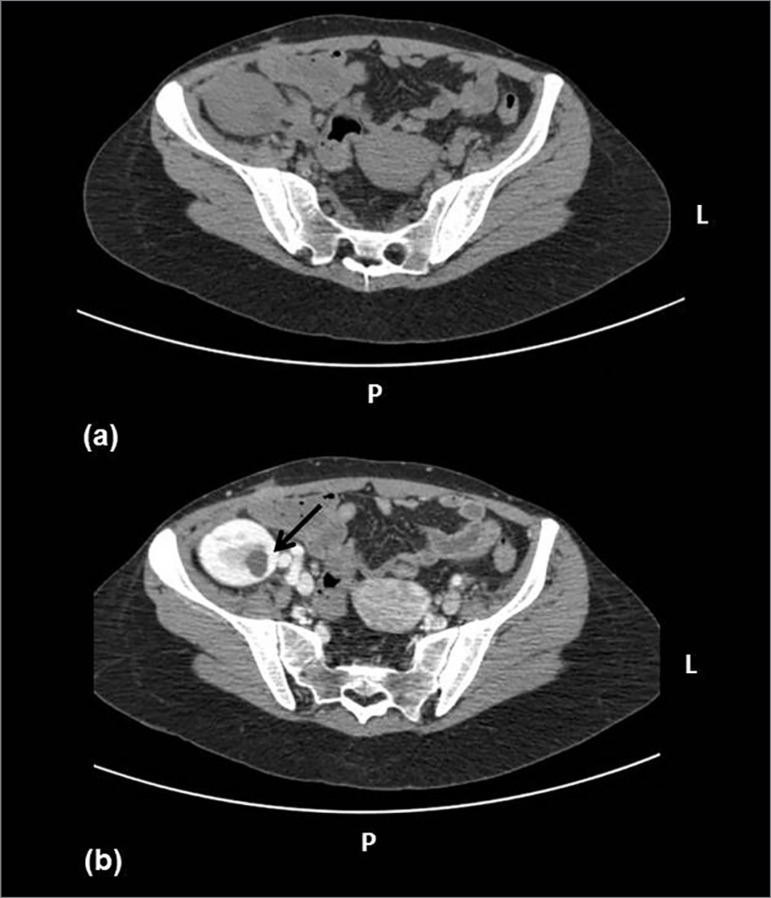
Panel A. Non-enhanced CT scan showing no attenuation area in the allograft. Panel B. Enhanced CT scan showing disclosed oval and wedge-shaped decreased enhancement areas, without cortical rim sign (outer cortical contrast uptake) in the upper third of the allograft (arrow).
